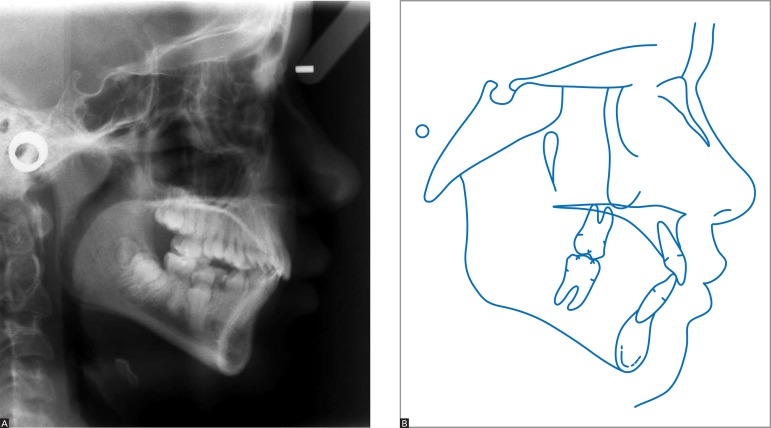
Intermediate profile cephalometric radiograph (A) and cephalometric
